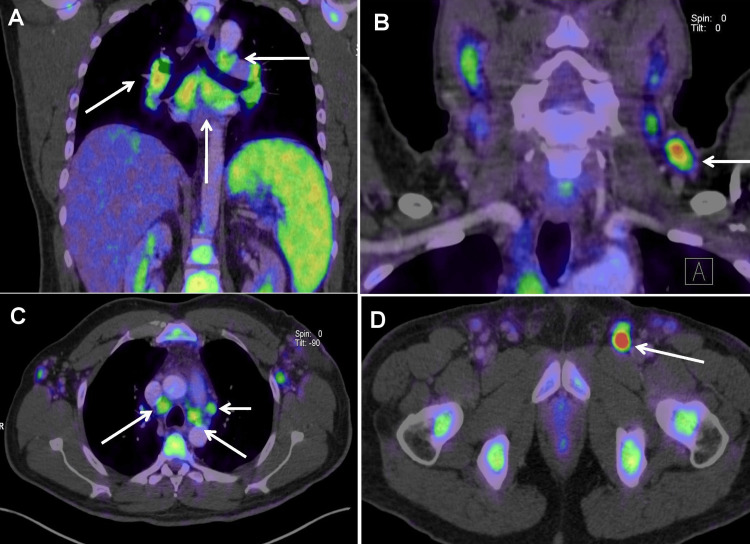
PET/CT showing multifocal lymphadenopathy (white arrows): (A) coronal plane with mediastinal and hilar involvement and splenomegaly; (B–D) axial planes showing cervical, paratracheal, paraaortic, axillary, and inguinal lymphadenopathy.
